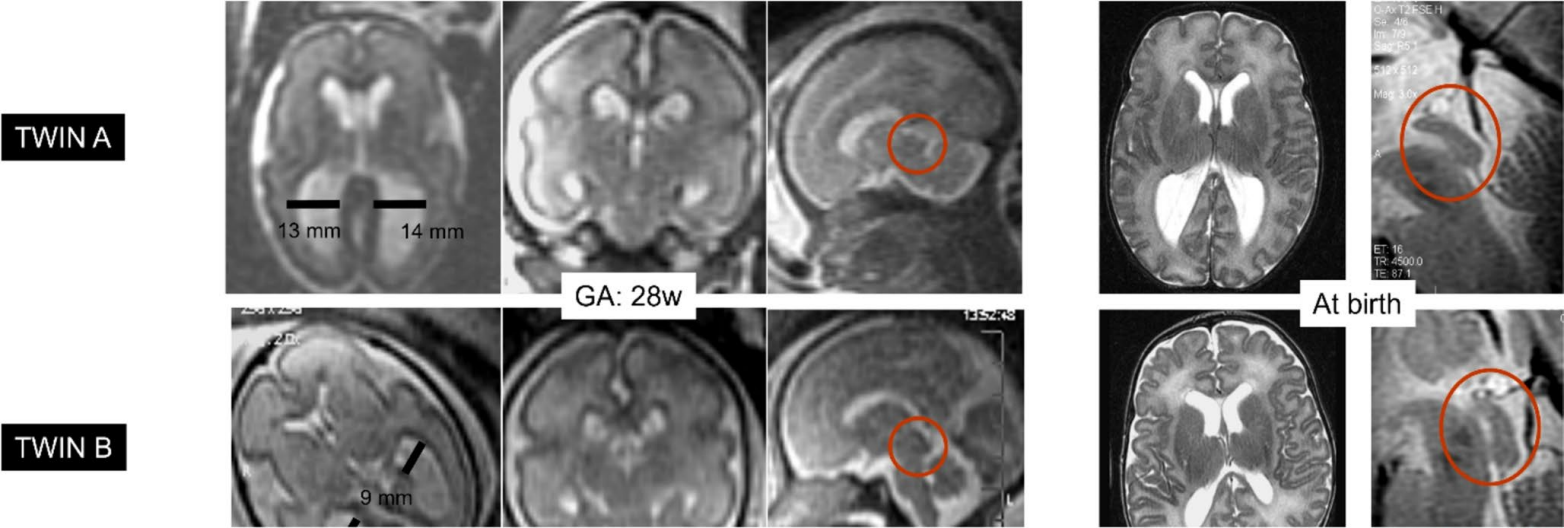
Monochorionic twins: an interesting paradigm of genetic-based aqueductal stenosis (autosomic recessive). Prenatal and postnatal T2-weighted images show stenosis of the lower third of the aqueduct, more evident in twin A, affected by moderate ventriculomegaly
